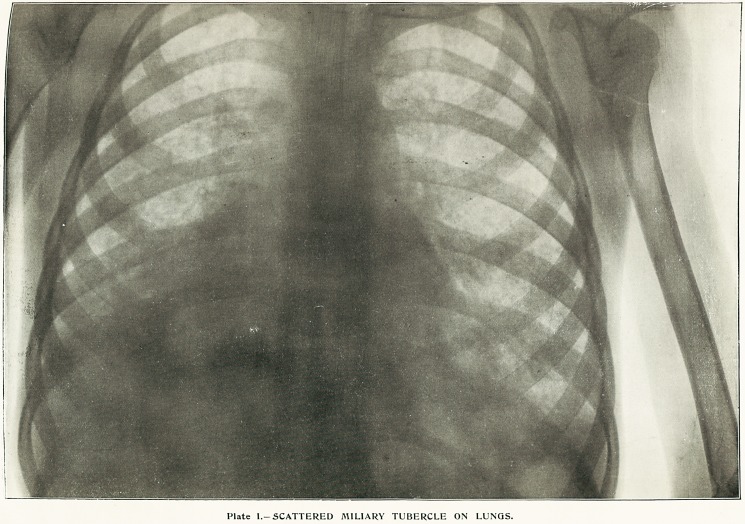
# Recent Progress in Radiography
